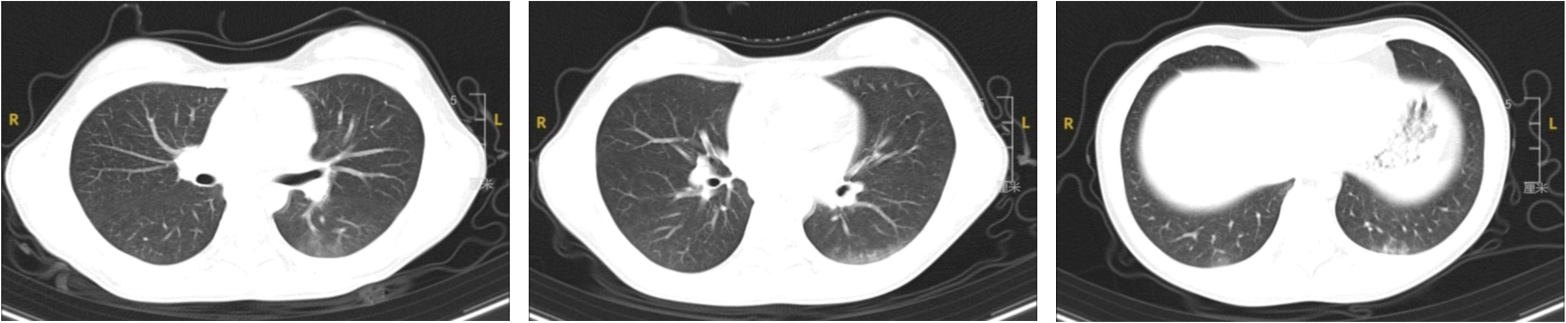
Chest CT findings of the patient.
